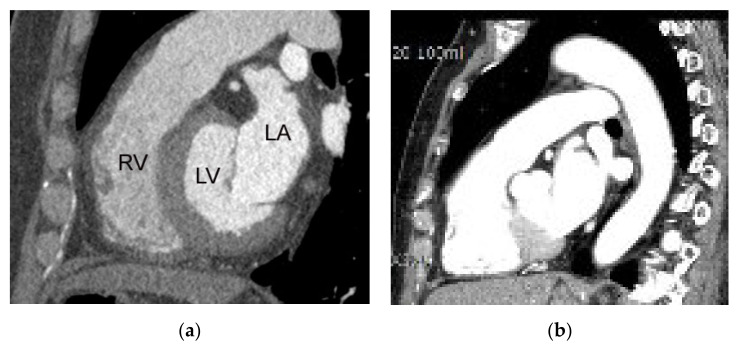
Typical z-axis coverage of dedicated coronary CTA versus triple-rule-out. (a) Z-axis coverage of dedicated coronary CTA includes the lower two-thirds of the entire chest. (b) The entire chest constitutes the z-axis coverage of the triple-rule-out CT. RV, LA, and LV indicate right ventricle, left atrium, and left ventricle, respectively.
